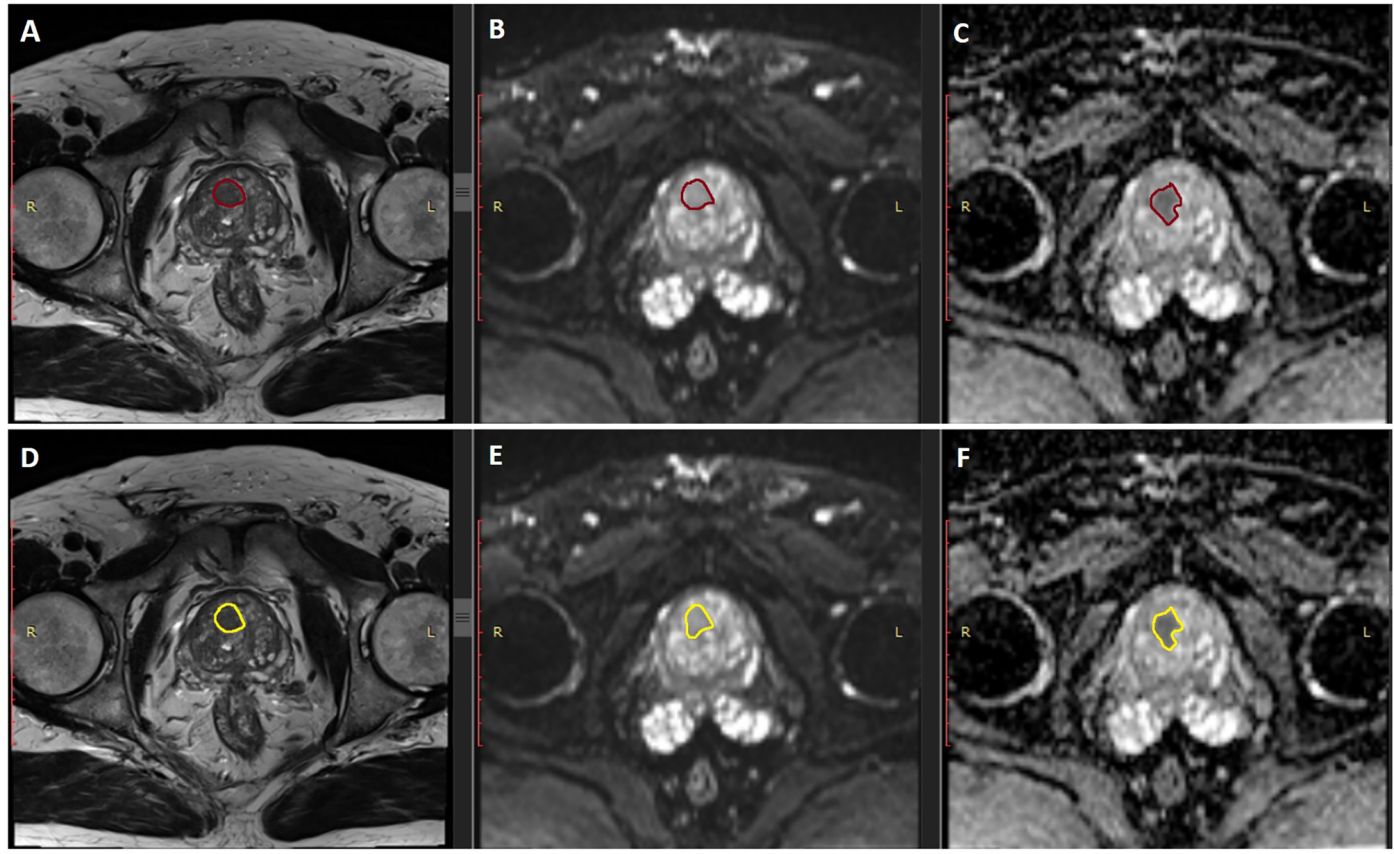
Examples of the two segmentation of the two operators. Panels (A–C) represent the examples of segmentation (in burgundy) of the first operator, in the T2, DWI. and ADC MRI sequences, respectively. Panels (D–F) represent the examples of segmentation (in yellow) of the second operator, in the same sequences, respectively.
